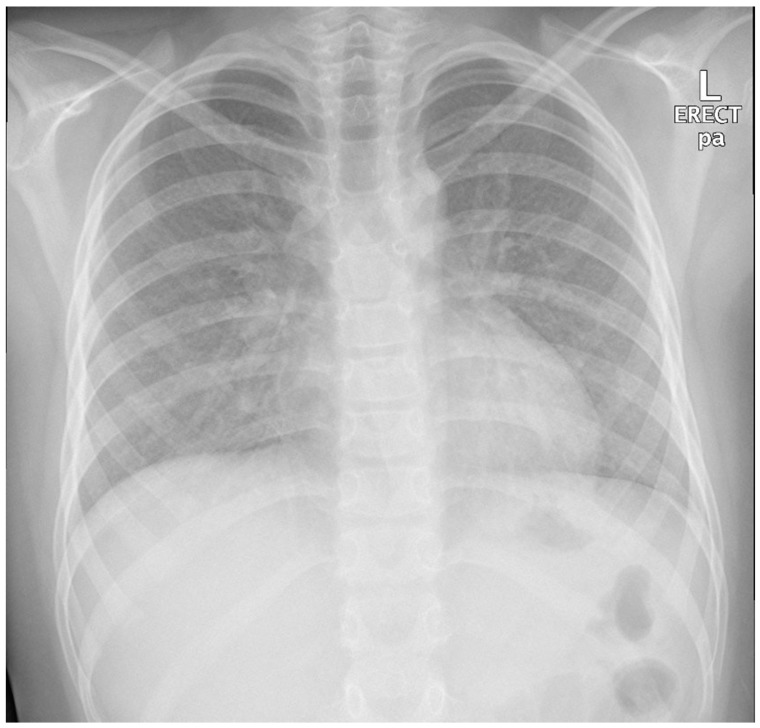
Patient first chest X-ray (CXR) showed bilateral diffuse miliary nodules.
